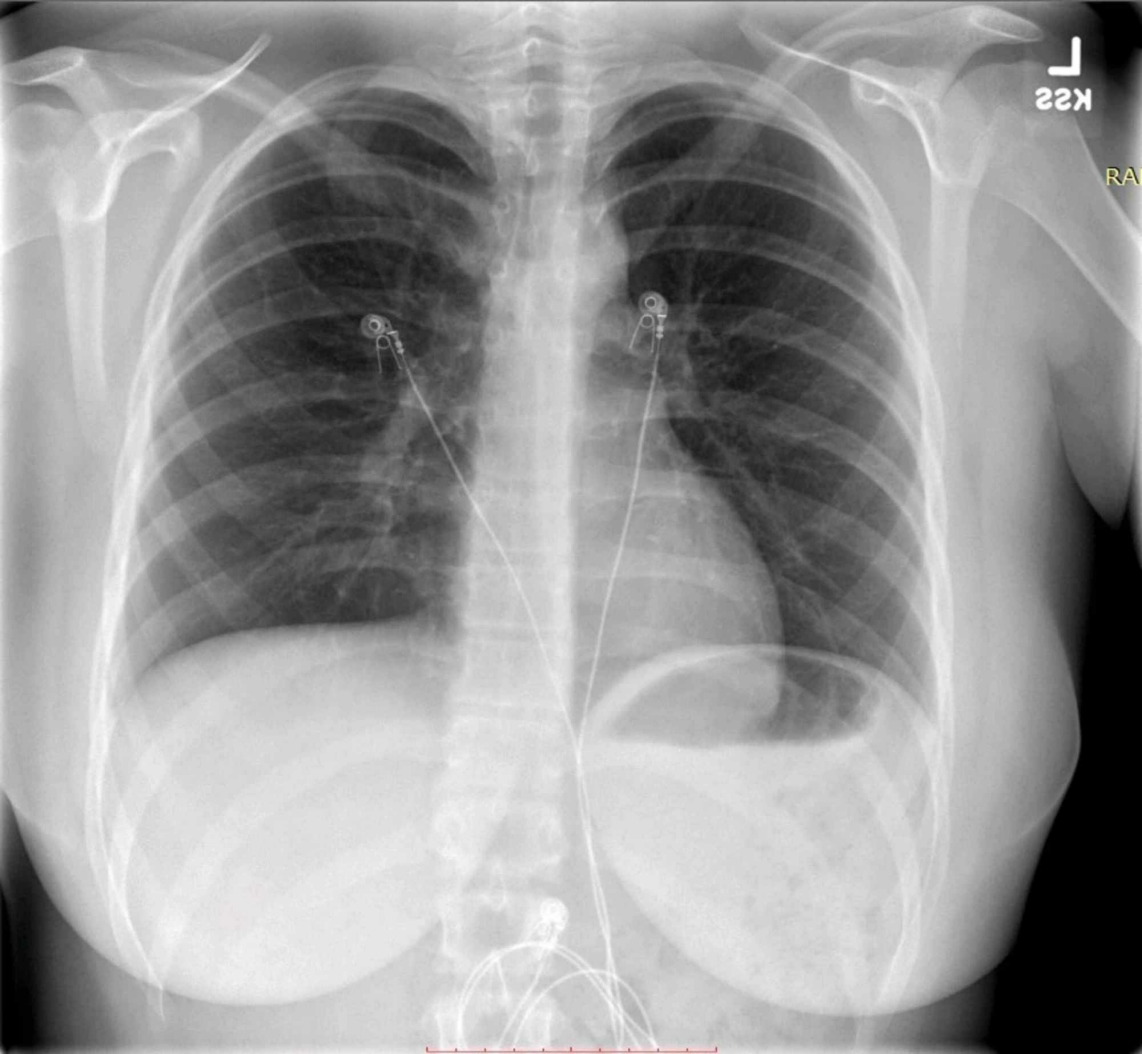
Two-view chest X-ray (anteroposterior view) showing no evidence of acute cardiopulmonary process
